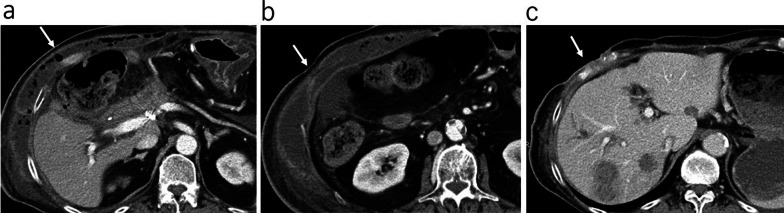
CT findings. a Contrast-enhanced CT showing necrotizing fasciitis and costochondritis of the right oblique abdominal muscle (arrow →). b Contrast-enhanced CT showing that the infected hematoma contacted to inner abdominal wall (arrow →). c Contrast-enhanced CT showing multiple liver metastases of 12 to 16 mm in diameter in both lobes. A subcutaneous abscess was detected on the right 11th rib (arrow →)
